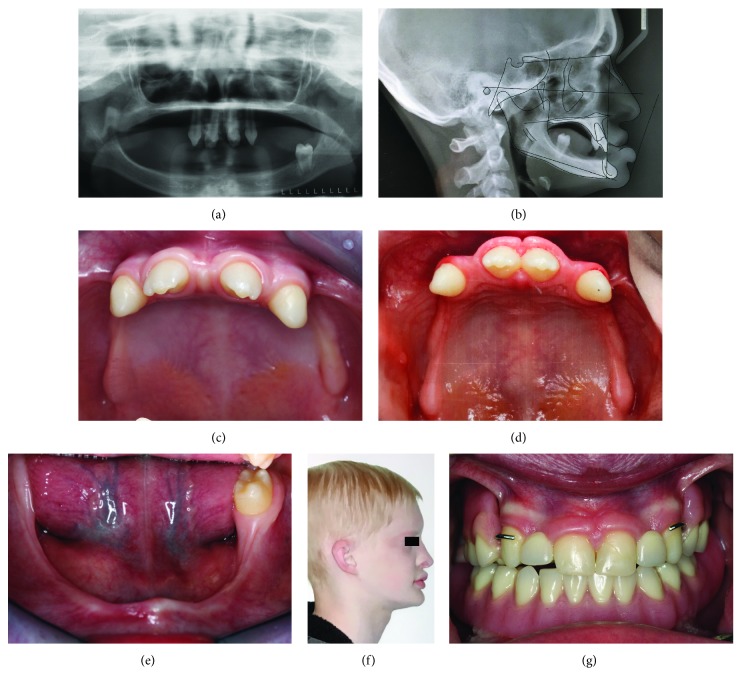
Clinic pictures of HED subject 1. (a) Panoramic radiograph at age twelve presented only five permanent teeth being developed on the patient. (b) Cephalometric radiography showed midface underdevelopment. (c) Patient presented with spacing teeth in the maxillary dentition before orthodontic treatment. (d) After orthodontic therapy (age fourteen), space between central incisors were closed. (e) Mandibular oligodontia was presented. Only left first molar was erupted. (f) Loss of vertical dimension of occlusion in profile view. (g) Front view of interim prosthesis in position.
